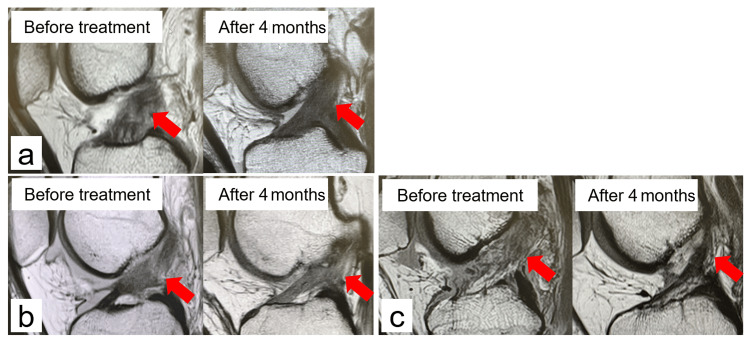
Repair condition grade assessed by MRI (a) Case 8: male 61 years old; the ACL, which was damaged and loose before treatment, regains continuity and tension four months later, and in addition, the ligament is considered to have a good grade 0 repair with low signal. (b) Case 1: female 21 years old; the ACL, which was torn before treatment, regained continuity after four months, and the repaired ACL was firm and thick but also showed high-signal areas and was considered to have a grade 1 repair. (c) Case 9: female 17 years old; the ACL, which was torn before treatment, regained continuity after 4 months, but the repaired ACL was thin and considered to have a grade 2 repair. ACL: anterior cruciate ligament
